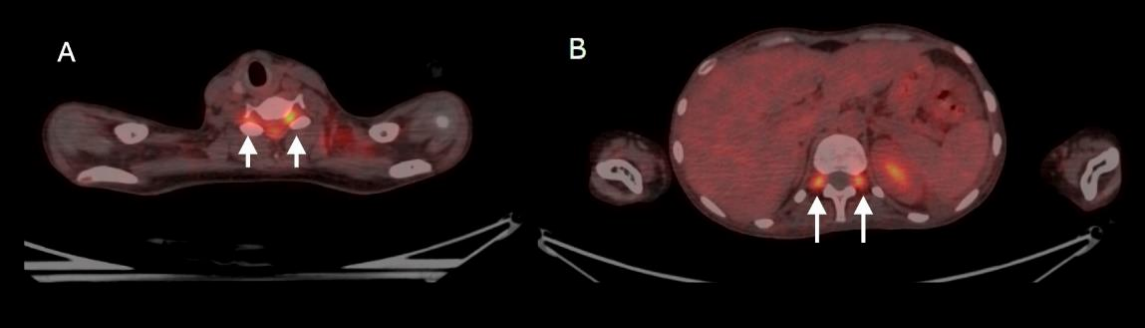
Transaxial fused PET/CT images at cervical level (A) and lumbar level (B) showing increased FDG uptake in the intervertebral foramina (white arrow)
